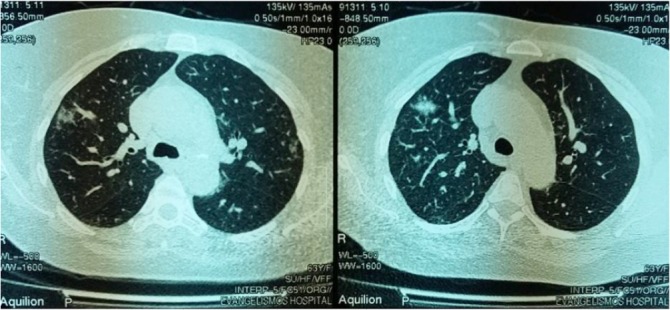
Chest CT scan showing a compact spindle – shaped mass in the right lower lobe surrounded by focal infiltration
